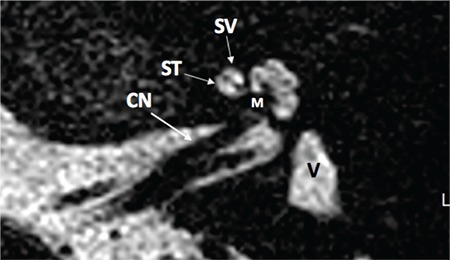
Axial T2 weighted magnetic resonance image showing cochlear nerve (CN), scala tympani (ST), scala vestibuli (SV), modiolus (M) and vestibule (V).
